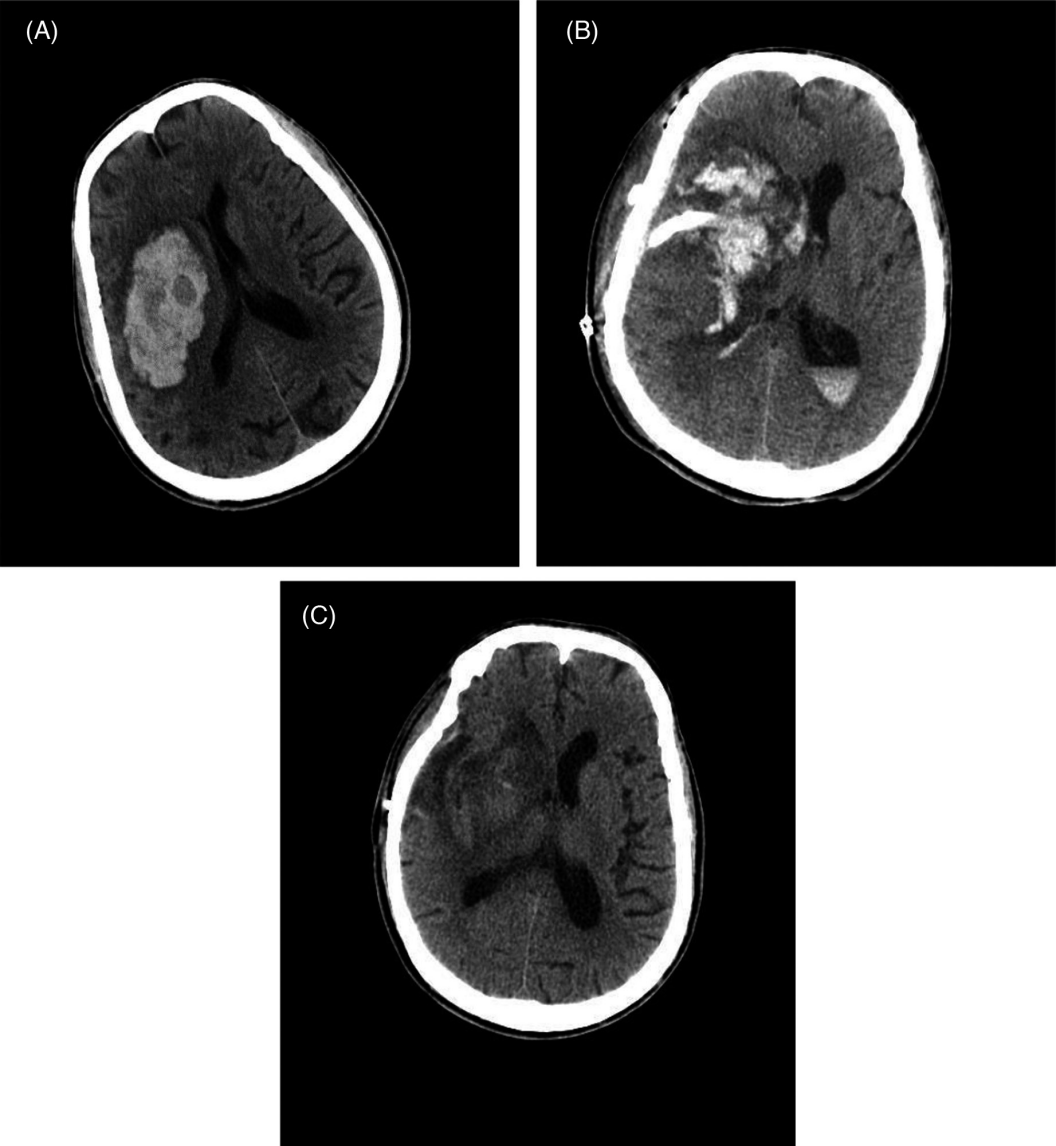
Cranial CT examination. Cranial (A), (B), and (C) CT images of the head showed preoperative, admission, and discharge intracranial conditions, respectively
